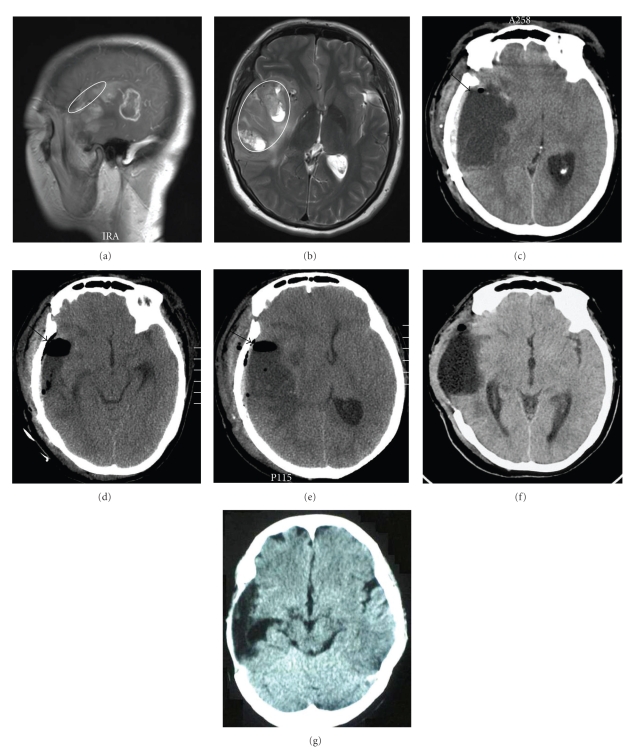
Imaging results for Case 1. (a) Contrast-enhanced MRI showing heterogeneous or ring-like enhancement in the temporal lobe. The elliptical area indicates the cortical incision, via which tumor resection was performed. (b) Axial T2WI showing the tumor resection zone. The cortical tissue located on the surface of the temporal lobe was not resected. (c) CT revealing cyst formation in the tumor resection cavity 3 days after tumor resection. The arrow indicates the position of the “valve”-like structure. (d) Relief of effusion in the tumor resection cavity following the reoperation. The arrow indicates the opening of the roof region of the tumor resection cavity and the disappearance of the “valve”-like structure. (e) Recommencement of effusion in the tumor resection cavity 5 days after the reoperation. The roof region of the tumor resection cavity was sealed again, indicating possible reformation of the “valve”-like structure. (f) Recommencement of effusion in the tumor cavity 1 week after the second reoperation removing the bone flap. (g) Disappearance of cyst formation in the tumor resection cavity 3 months after the second reoperation.
